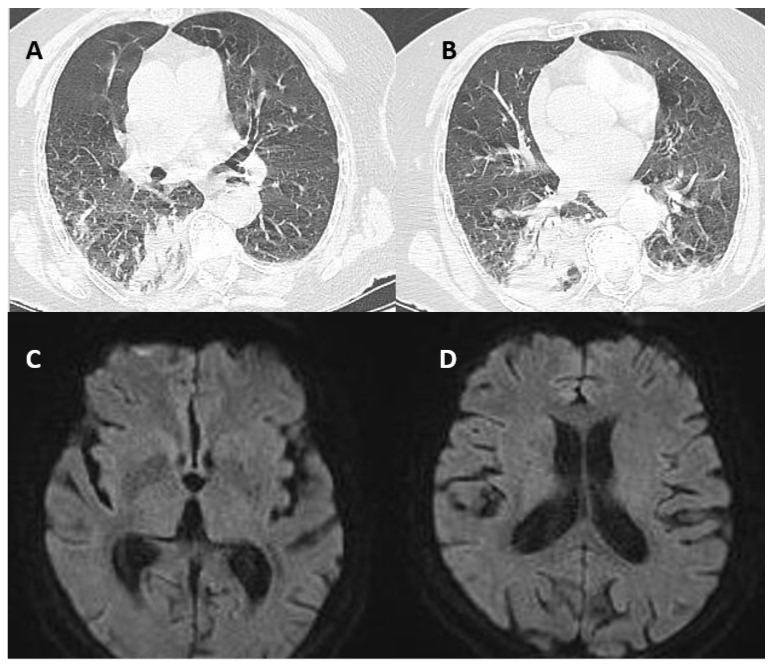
Imaging (A–D). Lung computed tomography shows bilateral areas of alveolar condensation and adjacent pleural effusion (A,B); Brain MRI-(DWI)—No areas of restricted diffusion were found (C,D).
